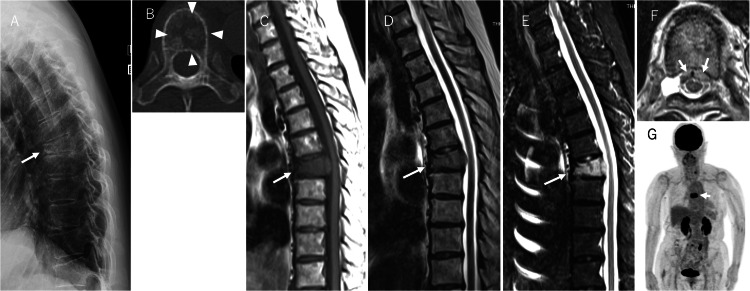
Imaging findings before MTX withdrawal. (A) Lateral view on X-ray at the first visit shows a reduction in the T7 vertebral body height and an increase in thoracic kyphosis (white arrow). (B) Axial view of the T7 vertebral body on CT shows osteolytic changes on the left side of the T7 vertebral body (white triangle). (C) Sagittal view on the T1-weighted MRI shows hypointense changes in the T7 vertebral body (white arrow). (D) Sagittal view on the T2-weighted MRI shows hypointense changes in the T7 vertebral body (white arrow). (E) Sagittal view of T2-weighted short-tau inversion-recovery (STIR) MRI shows hyperintense changes in the T7 vertebral body (white arrow). (F) Axial view of the T7 vertebral body on MRI shows the toxic twin-leaf sign around the posterior wall of T7 (white arrow). (G) Fluorine-18 fluorodeoxyglucose positron emission tomography/computed tomography (18F-FDG PET/CT) shows strong uptake in the T7 vertebral body (white arrow). MTX, methotrexate; CT, computed tomography; MRI, magnetic resonance imaging.
